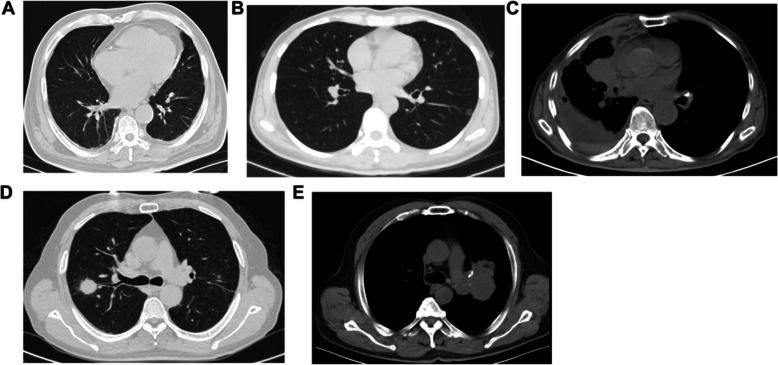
PET/CT imaging in patients with NSCLC. Pulmonary PET/CT in A normal individual, B patient with early adenocarcinoma, C patient with advanced adenocarcinoma, D patient with early squamous cell carcinoma, and E patient with advanced squamous cell carcinoma
